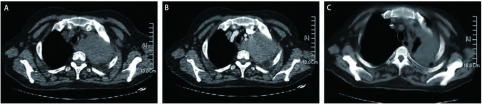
患者临床影像学特征。A：CT平扫：左上肺巨大肿块，侵犯纵膈；B：CT强化：肿块中度强化，包绕纵膈大血管，与食管分界不清；C：治疗后肿块明显缩小。 Clinical radiologic features of the patient. A: CT scan: a huge mass in the left upper lung invaded the mediastinum; B: CT contrast enhanced scan: mass medium sclerosis surrounded great vessels in the mediastinum, and no clear boundary with esophaged; C: the mass shrunk significantly after chemotherapy.
